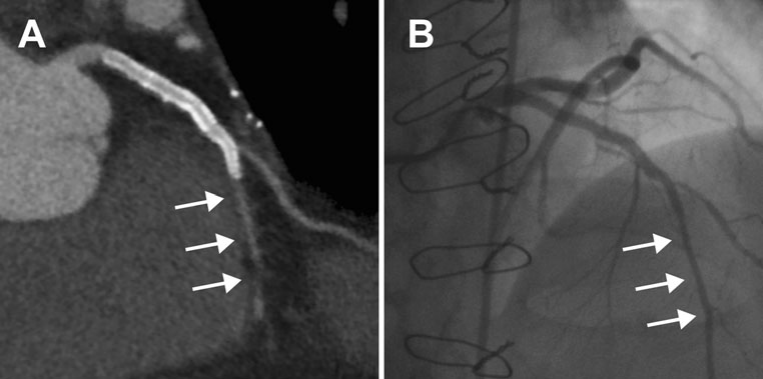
Example of a false positive CTA from a 54 year old male with atypical chest pain, known CAD treated with single vessel bypass surgery to the left anterior descending (LAD) followed a proximal LAD stent (3.0 mm diameter) 6 years later due to graft occlusion. The CTA had poor contrast opacification of the LAD (a, *arrows*) beyond the stent which was interpreted as a severe stenosis. This finding was confirmed on axial and multi-planar reformat imaging. Invasive angiography demonstrated a patent proximal LAD stent with good opacification of the distal vessel (b, *arrows*). CMR demonstrated normal stress perfusion and no evidence of myocardial infarction (not shown)
